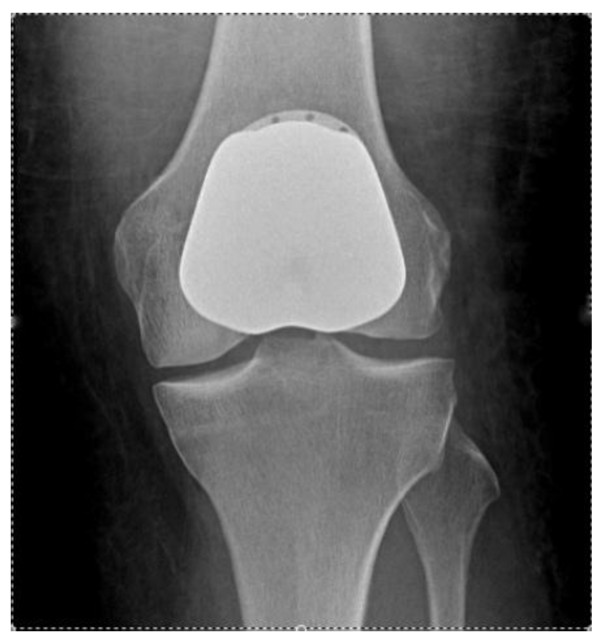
AP radiograph showing a Patellofemoral Arthroplasty.
